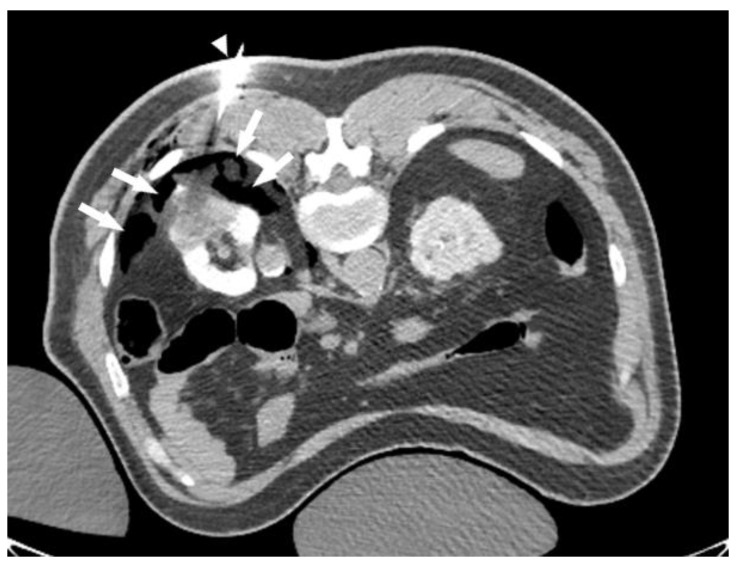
CO_2_ dissection. CT scan of the same patient in Figure 1 during ablation. CO_2_ has been injected through a 21 g needle (white triangle). Notice that CO_2_ (arrows) accumulates in the most posterior, non-dependent, fat planes, creating an insulating plane between the kidney and intercostal nerves.
